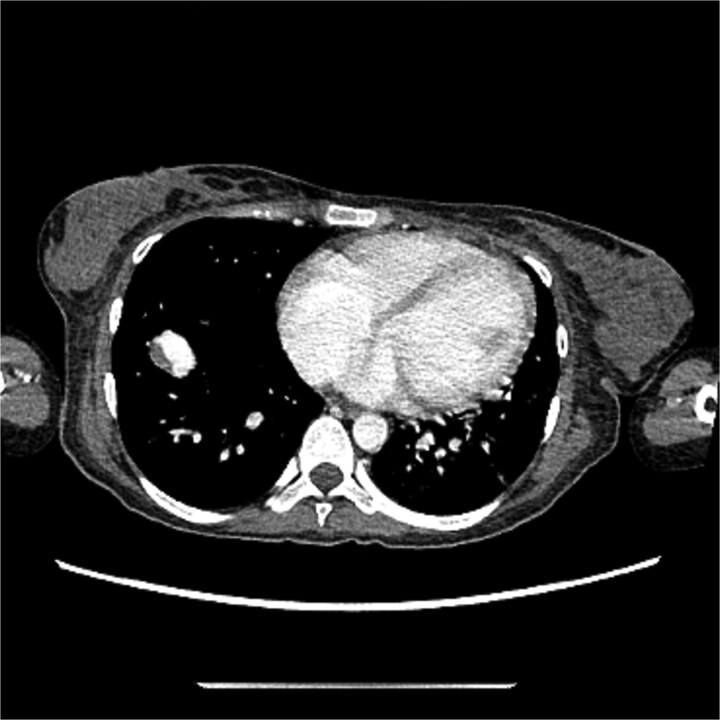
CT of the chest showed multiple AVMs.
